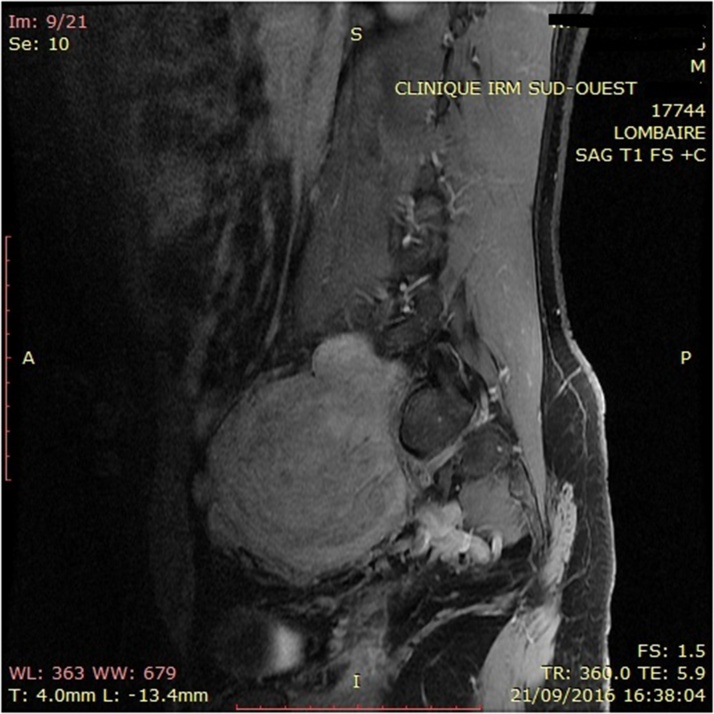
Magnetic Resonance Imaging showing an encapsulated and well limited retro peritoneal masse.
